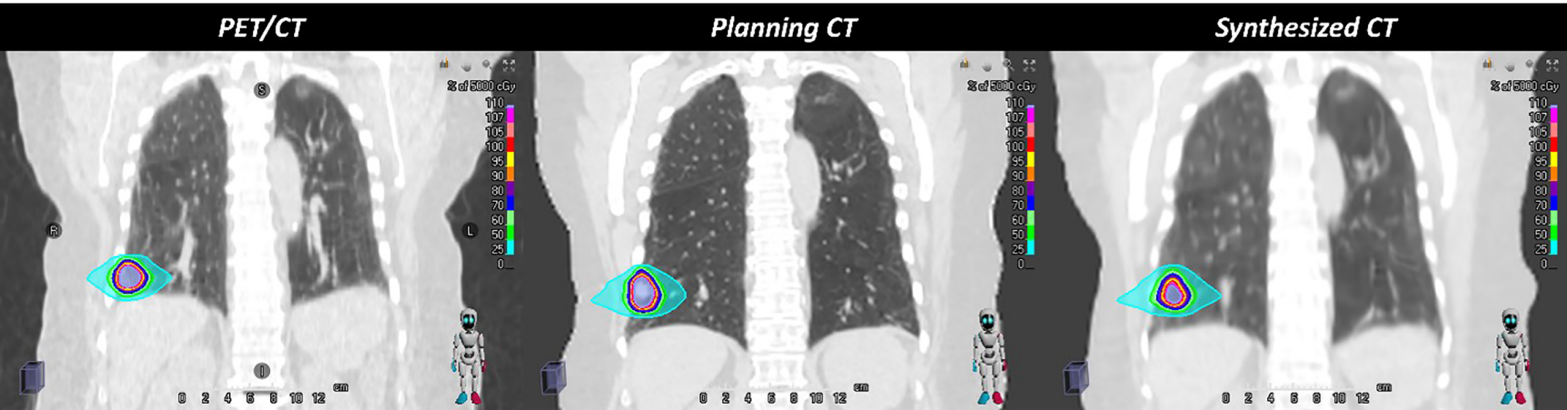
The figure demonstrates the anatomical differences due to the breath-hold motion management technique, which causes a large dosimetric difference between the RT plans recalculated on planning CT and PET/CT scans. However, the deepPERFECT synthesized CT scans captured the anatomical changes and the dose difference between the planning CT and synthesized CT was significantly reduced. Therefore, PET/CT cannot be directly used in treatment planning.
